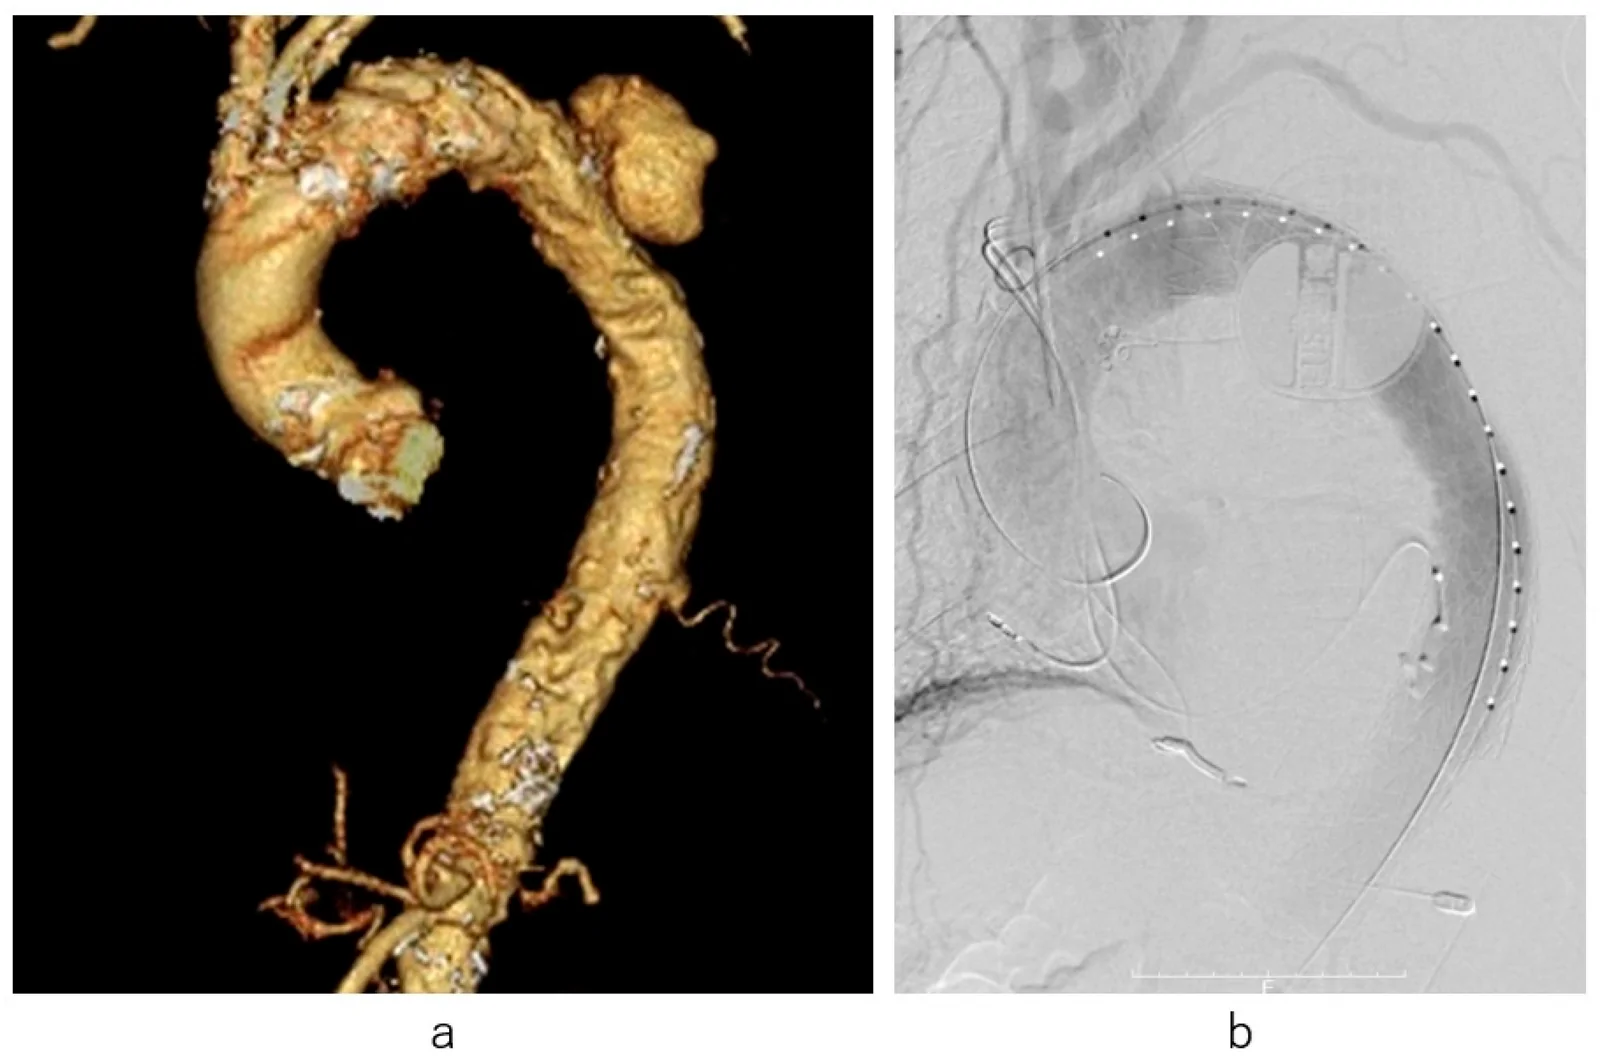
Recurrent rupture and subsequent thoracic endovascular aortic repair. (a) Three-dimensional computed tomography after recurrent hemoptysis showed further enlargement of the ulcer-like projection and descending thoracic aorta. A new aortobronchial fistula involving the peripheral bronchus of the left lower lobe was suspected. (b) Completion angiography after thoracic endovascular aortic repair showed exclusion of the aortic lesion without endoleak.
